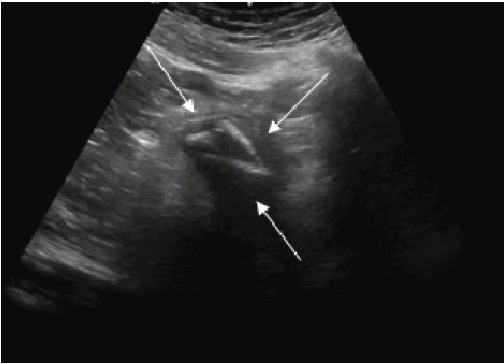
Sagittal ultrasound view of the bladder using a curvilinear probe. Three hyperechoic foreign bodies (arrows) are seen in the bladder.
